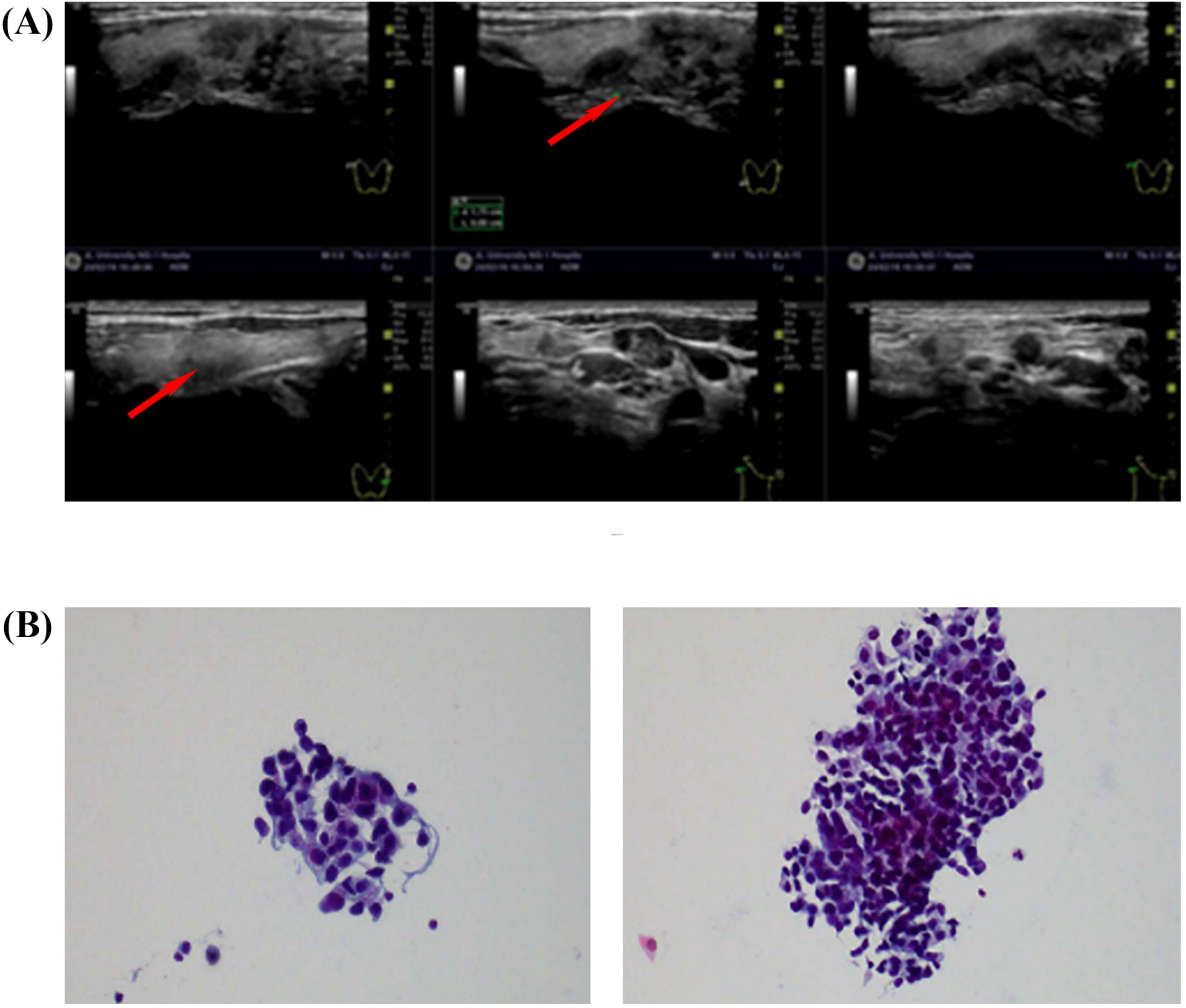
(A) Thyroid and regional lymph node color Doppler ultrasound (19 February 2024). (B) FNAC of thyroid and lymph node lesions. Smear from the right thyroid lobe (left). Aspirate from a right cervical level IV lymph node (right).
